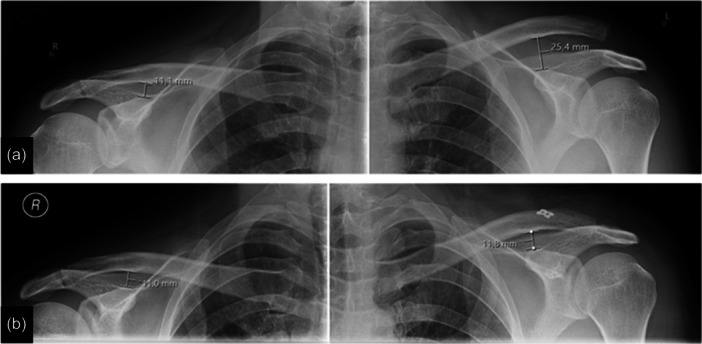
(a) Preoperative radiograph of a patient with an acute Rockwood V acromioclavicular joint (ACJ) left shoulder injury. (b) Arthroscopically assisted anatomic acromioclavicular and coracoclavicular stabilisation postoperative radiograph.
